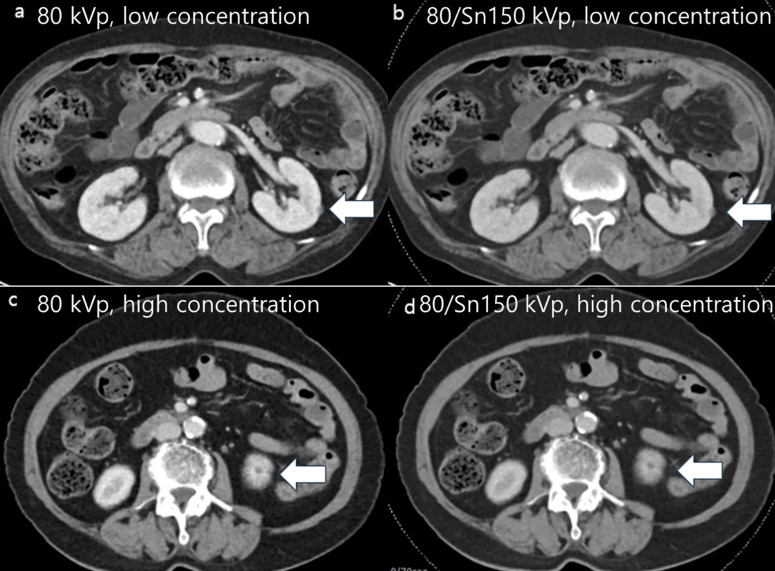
Abdominopelvic computed tomography (CT) images of patients with bladder and breast cancers. CT images of a patient with bladder cancer (76-year-old, female; weight, 59.3 kg; body mass index, 25.0 kg/m^2^; a-b) and a patient with breast cancer (80-year-old, female; weight, 55 kg; body mass index, 25.8 kg/m^2^; c-d) with matching cases. (a-b) CT images show a left renal cyst (5 mm in size) using a low concentration of contrast agent and (c-d) a left renal cyst (4 mm in size) using a high concentration of contrast agent. The overall image quality score was 2 for the 80 kVp CT image (a), 3 for the 80/Sn 150 kVp image (b), 2 for the 80 kVp CT image (c), and 3 for the 80/Sn 150 kVp image (d). The renal lesion conspicuity and margin sharpness were similar, showing a score of 3 for conspicuity in all images (a-d), a score of 3 for margin sharpness in images (a) and (c), and a score of 4 in images (b) and (d).
